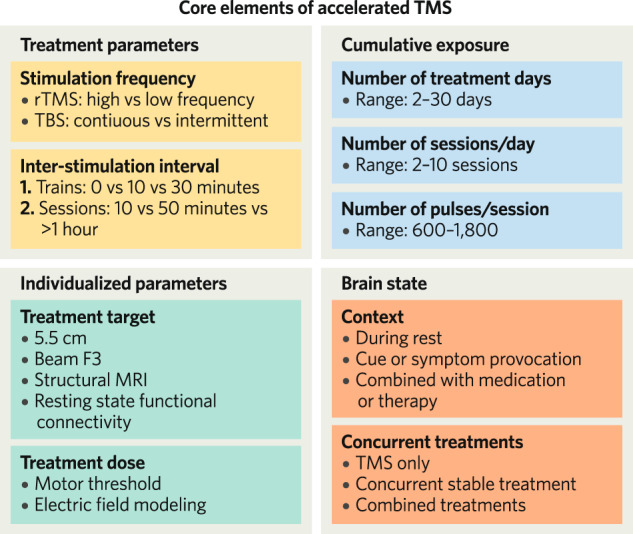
Core elements of accelerated TMS. The nine core elements considered in this review for accelerated TMS. rTMS repetitive transcranial magnetic stimulation, TBS theta-burst stimulation, MRI magnetic resonance imaging.
